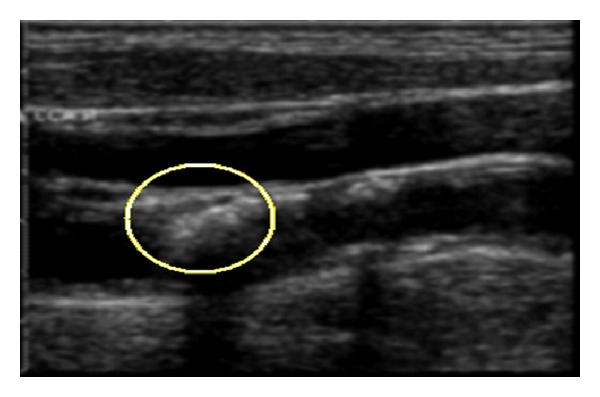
Carotid artery ultrasound image. The drawn ellipse marks a stenosis.
